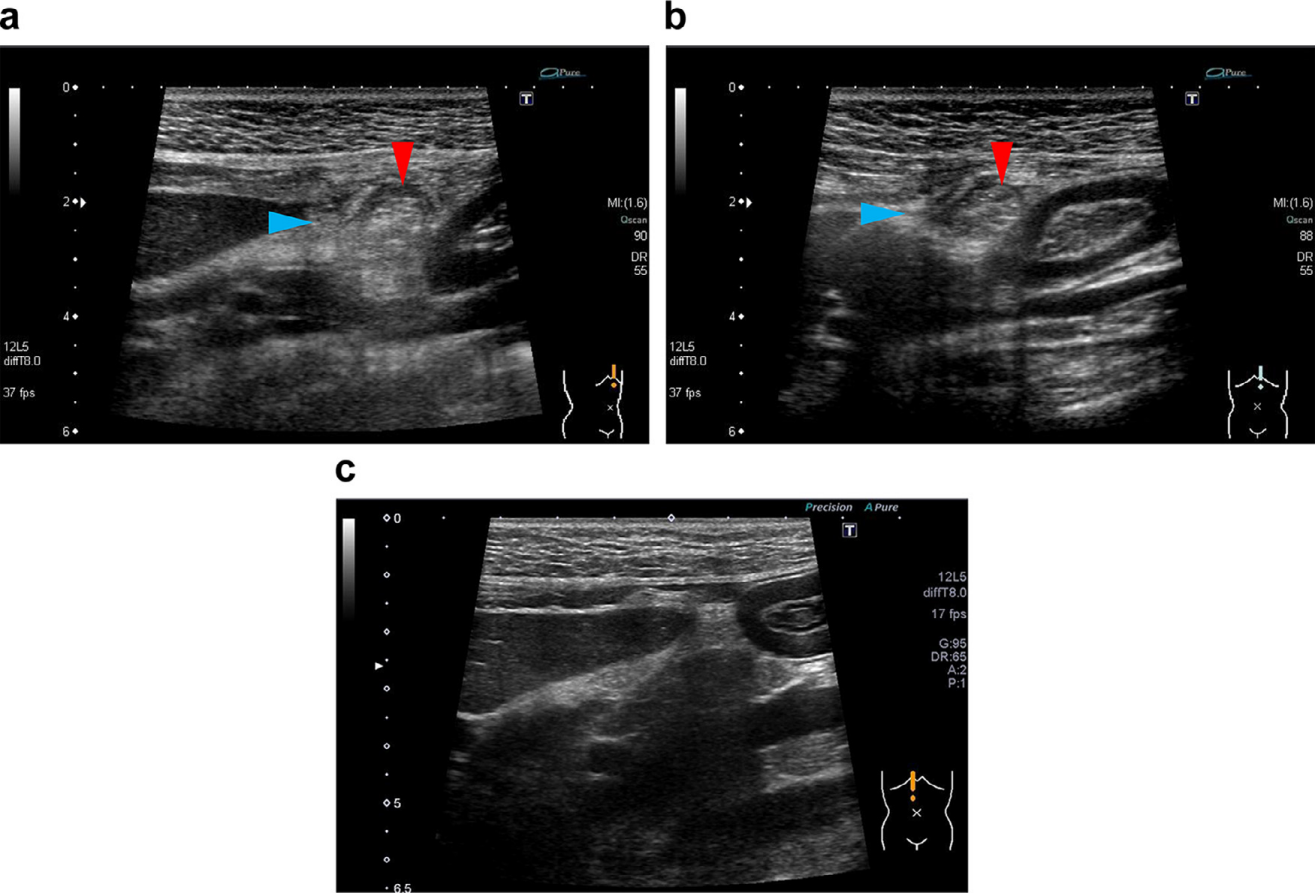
Changes in the ultrasound findings of falciform ligament appendagitis on follow-up of Case 2. (A) At the time of onset, there is an oval hyperechoic mass with a peripheral hypoechoic rim (red arrow head) and increased echogenicity of surrounding fat (blue arrow head). (B) One week later, the mass has shrunk remarkably (red arrow head) and the surrounding inflammatory fat is reduced (blue arrow head). (C) Eight months later, there is complete resolution of the hyperechoic mass and surrounding inflammatory fat.
